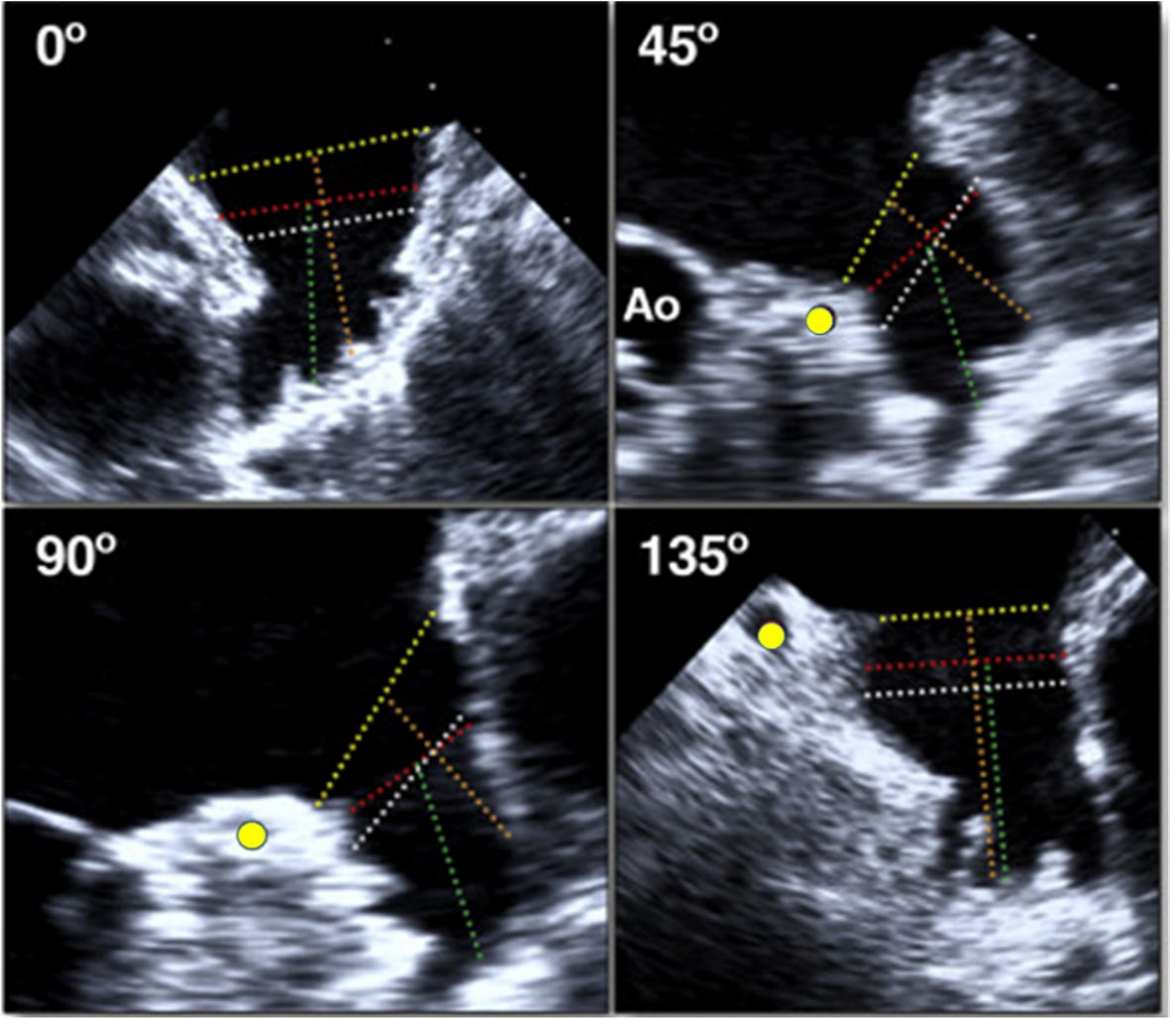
Scan of the left atrial appendage from 0°, 45°, 90°, and 135° views using two-dimensional transthoracic echocardiography to measure the maximum orifice diameter, landing zone, and maximum depth before device closure. Yellow circle indicates the circumflex artery
